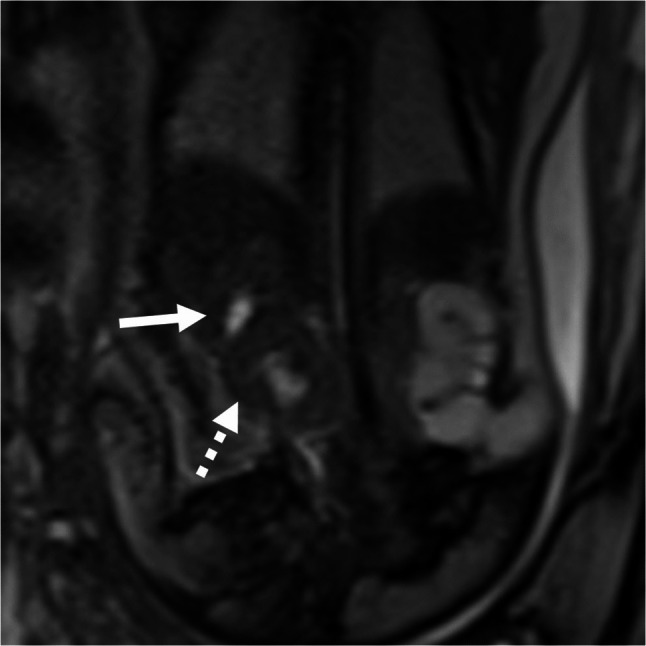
Coronal Single-Shot Fast Spin Echo (SSFSE) MRI of a 29-week gestation male fetus showing a left-to-right crossed fused renal ectopia (right - arrow, left - dotted arrow). The right and left renal moieties are positioned at 90° and both are located in the right hemiabdomen
